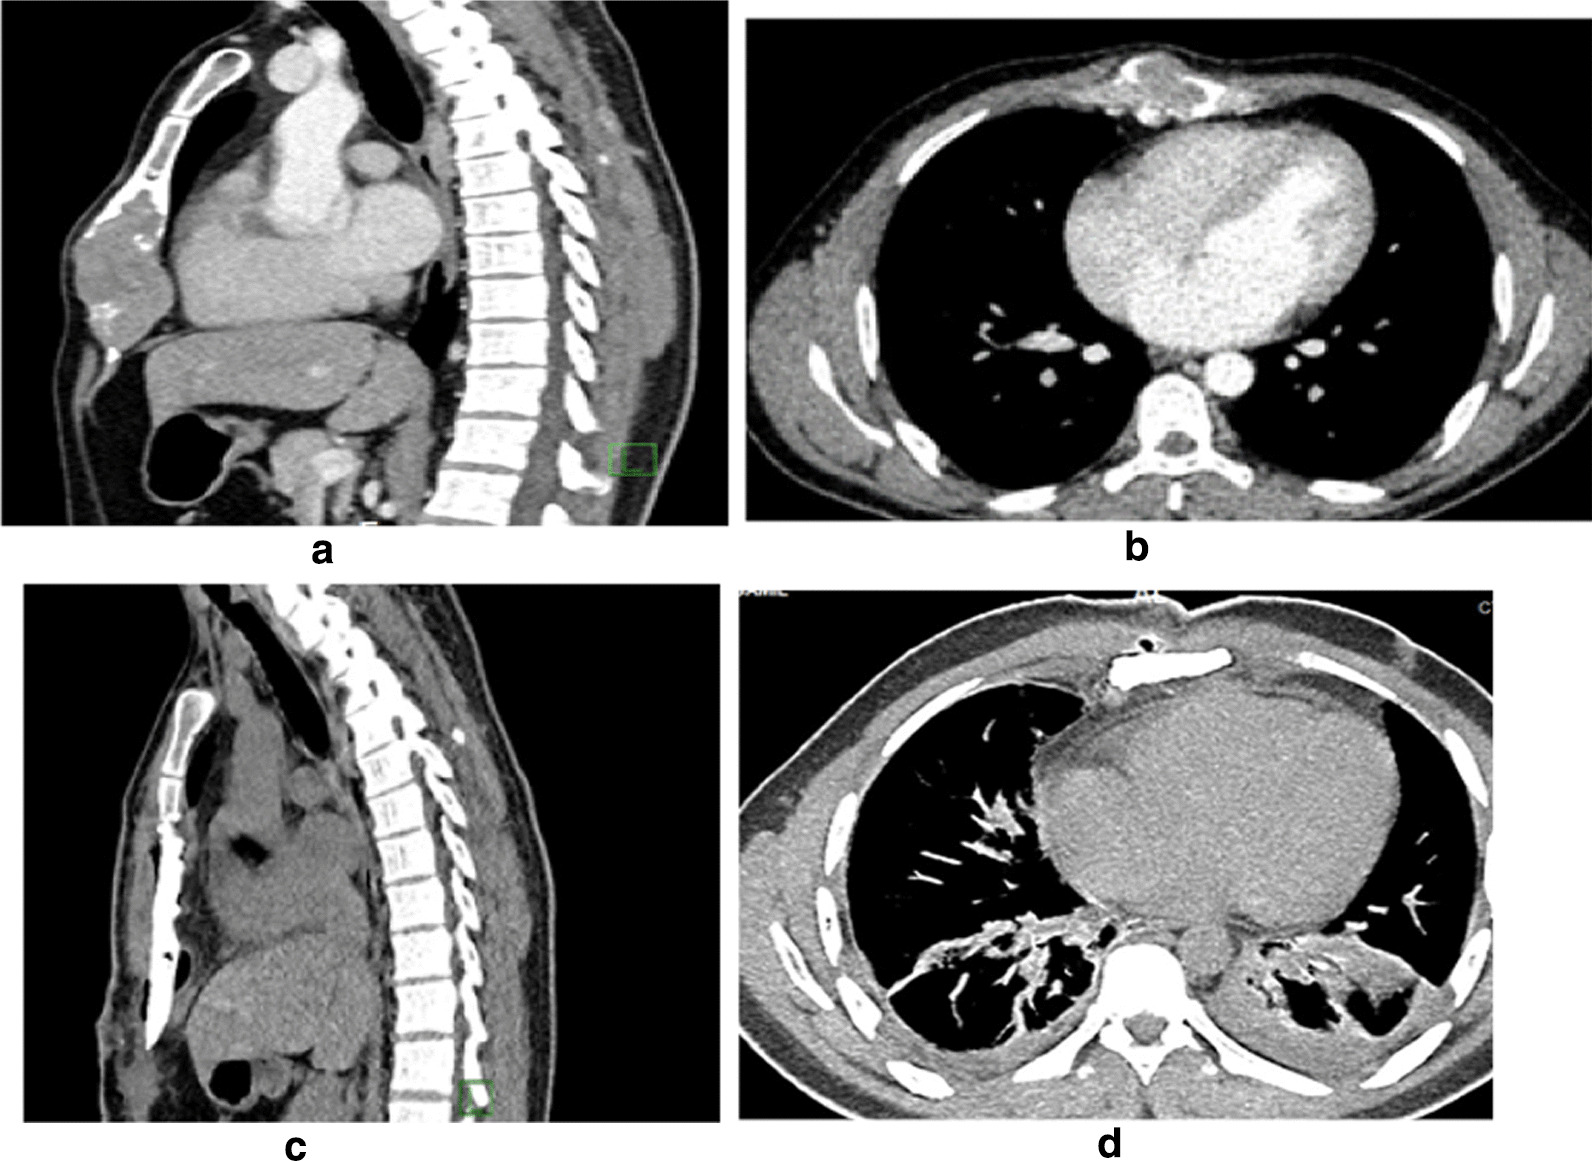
CT Chest mediastinal window: sagittal (a) and axial, (b) mediastinal window: showed large, expanding lytic lesion in the lower two-thirds of the sternum. CT Chest mediastinal window, c Sagittal midline sternum displayed post- operative after lower sternal tumor resection and reconstruction with replacement with prosthetic material. d Axial mediastinal window: bilateral mild pleural effusions with consolidation collapse of the lower lobes and subsegmental atelectasis. Air bubbles in the sternal surgical bed and subcutaneous edema without gross residual masses or localized fluid collections
